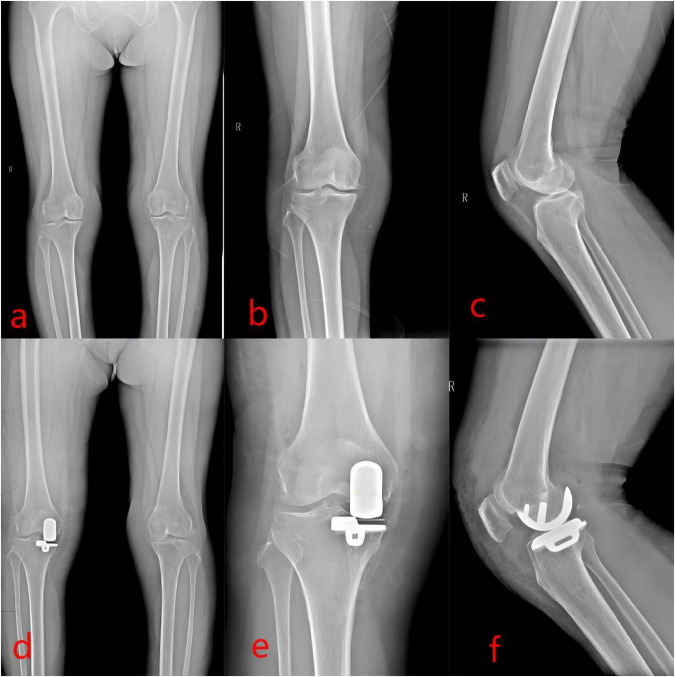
Unicompartmental knee arthroplasty (UKA). This figure shows a 65-year-old female patient. (a–c) Preoperative anteroposterior and lateral X-rays of the right knee joint and full-length X-rays of the lower extremity demonstrate the manifestations of knee osteoarthritis (KOA) in the right knee joint, with narrowing of the medial compartment and mild varus deformity of the knee joint. (d–f) Postoperative anteroposterior and lateral X-rays of the right knee joint and full-length X-rays of the lower extremity show that the prosthesis is well fixed without any sign of loosening. The varus deformity and narrowing of the knee joint have been corrected, and the mechanical axis of the lower extremity has been significantly improved compared with that before the operation.
